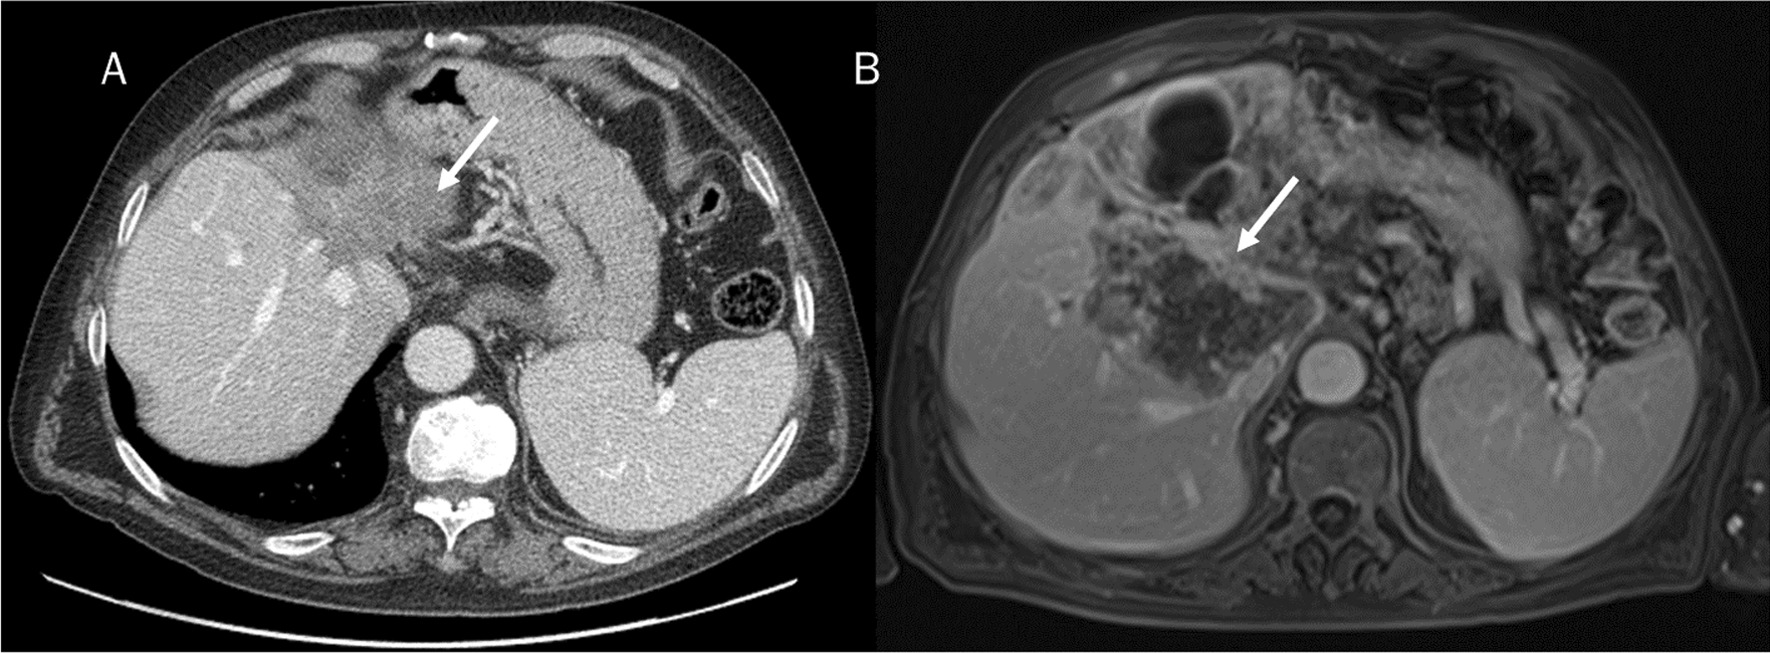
ICC on II-IV hepatic segment assessed by CT (A) and MRI (B). The lesion shows peripheral rim hyperenhancement with progressive contrast enhancement (arrow)
